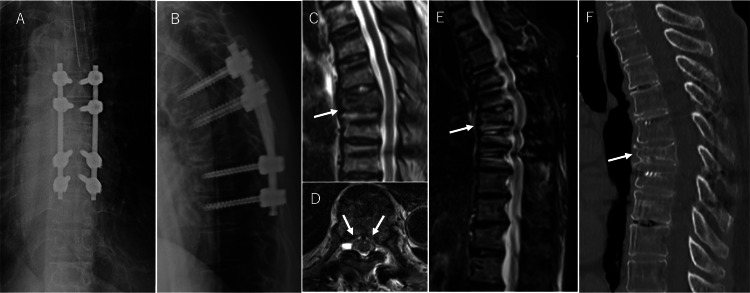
Imaging findings after MTX withdrawal and posterior fusion of the thoracic spine. (A) Anteroposterior (A-P) view on postoperative X-ray shows the thoracic posterior fusion with spinal instrumentation from T5 to T9. (B) Lateral view on postoperative X-ray shows the posterior fusion with spinal instrumentation from T5 to T9. (C) Sagittal view on T2-weighted MRI two weeks after MTX withdrawal shows tumour regression of the T7 vertebra (white arrow). (D) Axial view of the T7 vertebral body on MRI shows that the toxic twin-leaf sign had almost disappeared around the posterior wall of the T7 vertebra (white arrow). (E) Sagittal view on MRI (STIR) 30 months after MTX withdrawal shows that the tumoral lesion remains obliterated (white arrow). (F) Sagittal view on CT scan 30 months after MTX withdrawal shows a solid union of T7 vertebra, bridging to the caudal vertebrae (white arrow). MTX, methotrexate; MRI, magnetic resonance imaging; CT, computed tomography.
